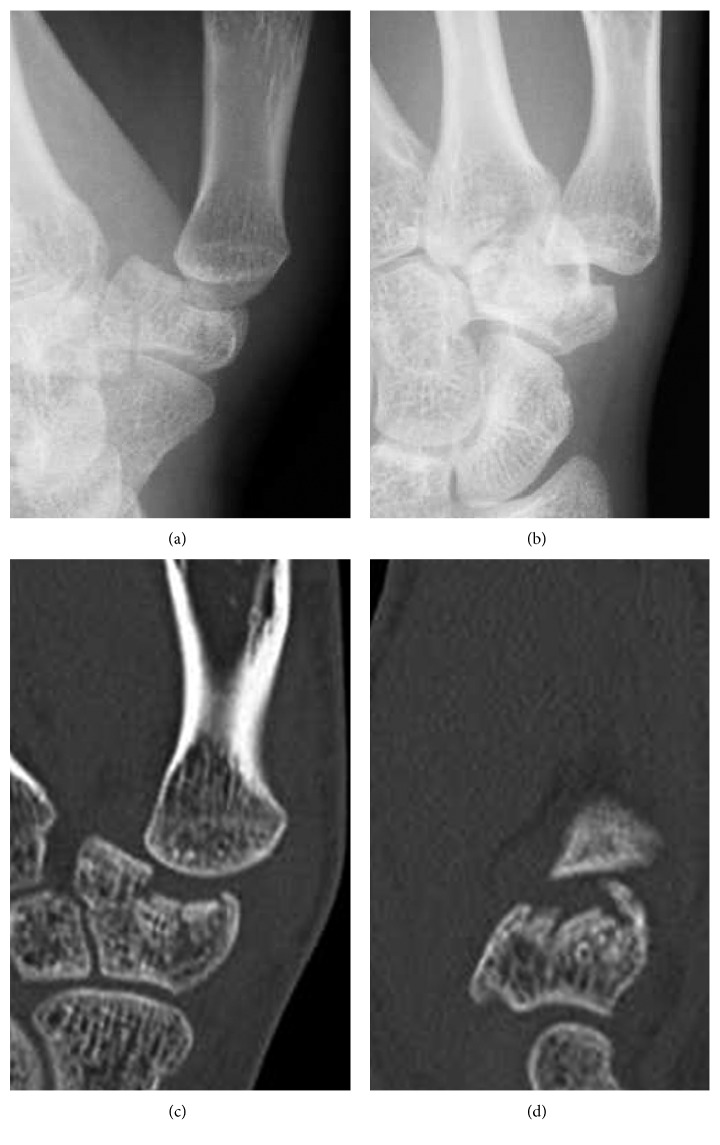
(a, b) Radiographs taken at the initial presentation. The trapezium body fracture and radial dislocation of the CMC joint are shown. (c, d) Computed tomography taken at the initial presentation. The collapse of the trapezium articular surface is shown. (a, c: anteroposterior view; b, d: lateral view.)
